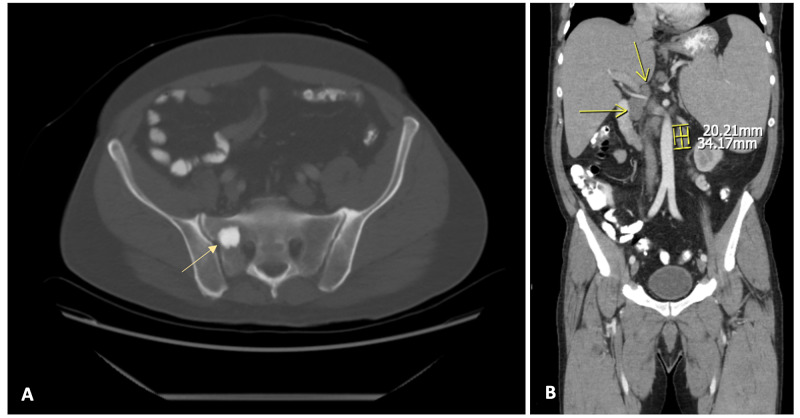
An axial image (A) from a CT pelvis shows an increased signal in the right sacrum and the coronal image (B) showing periportal and retroperitoneal lymph nodes suspicious for lymphoid neoplasm.
